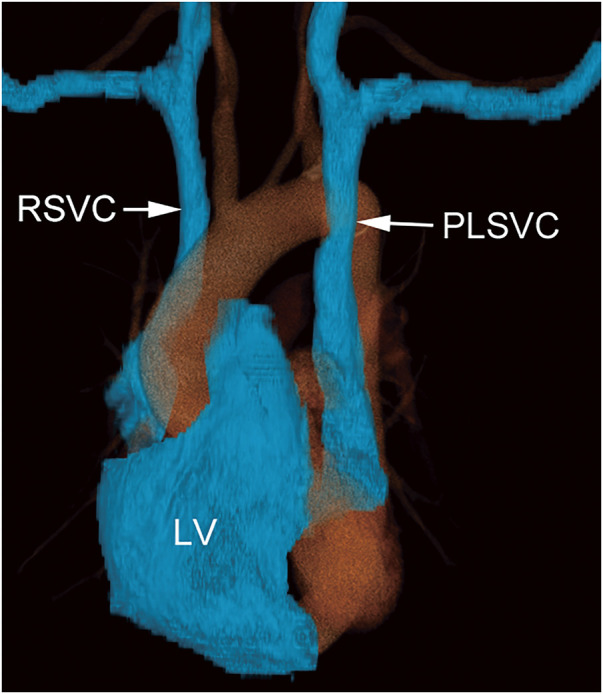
Three-dimensional reconstruction of the computed tomography image. The three-dimensional view of a CT scan from the anterior left side shows the relationship between the heart and the PLSVC. RSVC, right superior vena cava; PLSVC, persistent left superior vena cava; LV, left ventricle
